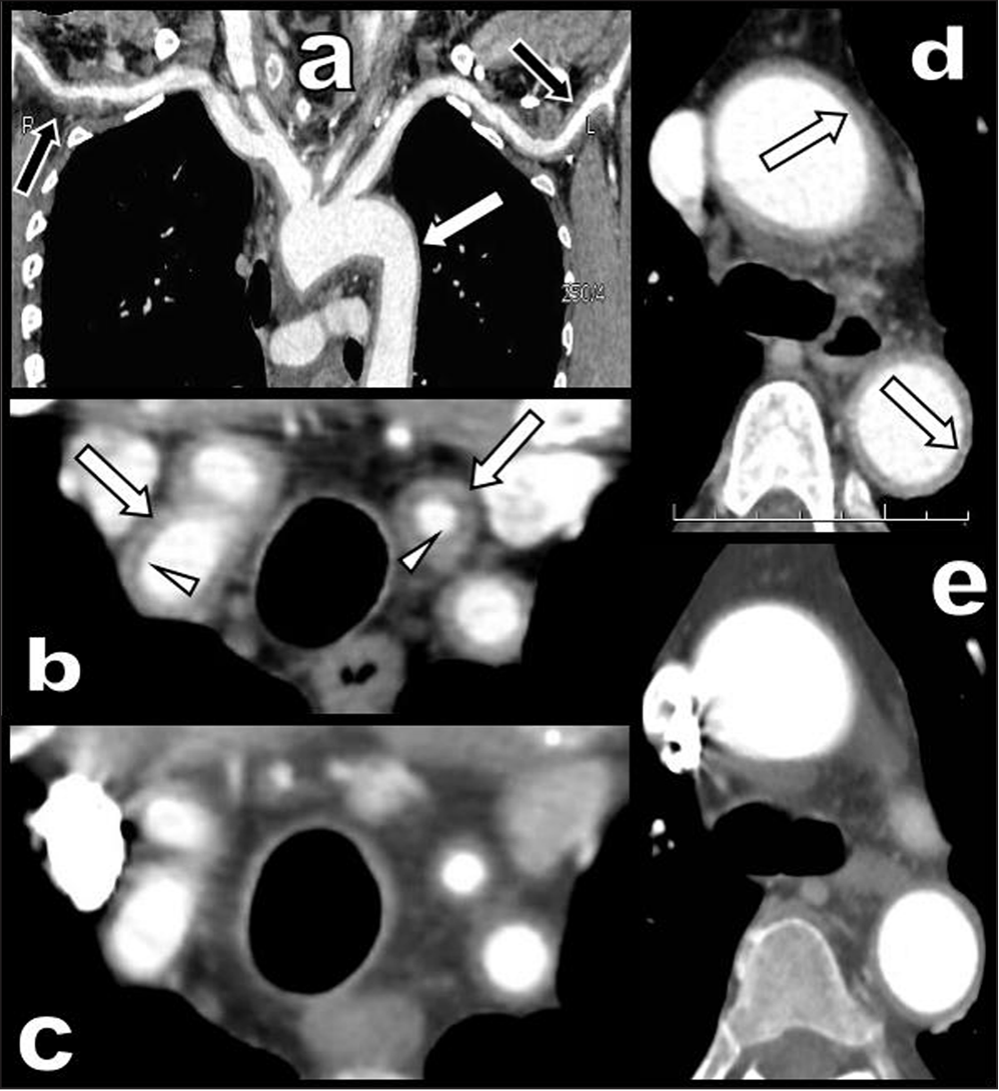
CT angiography of the aortic arch and of its large emerging arteries before (a, b, d) and after treatment (c and e).
